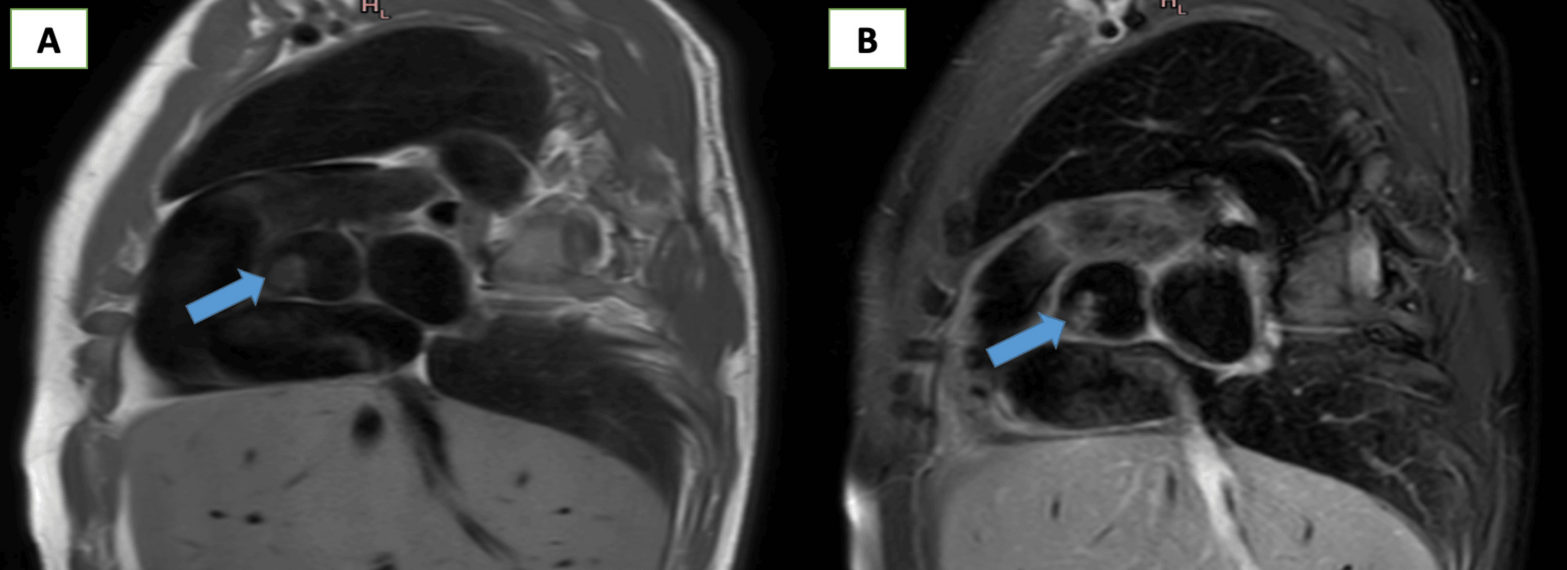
CMR images (A) showing an iso-intense signal of the mass in the T1-weighted image and (B) showing a hyper-intense signal of the mass in the T2-weighted image
